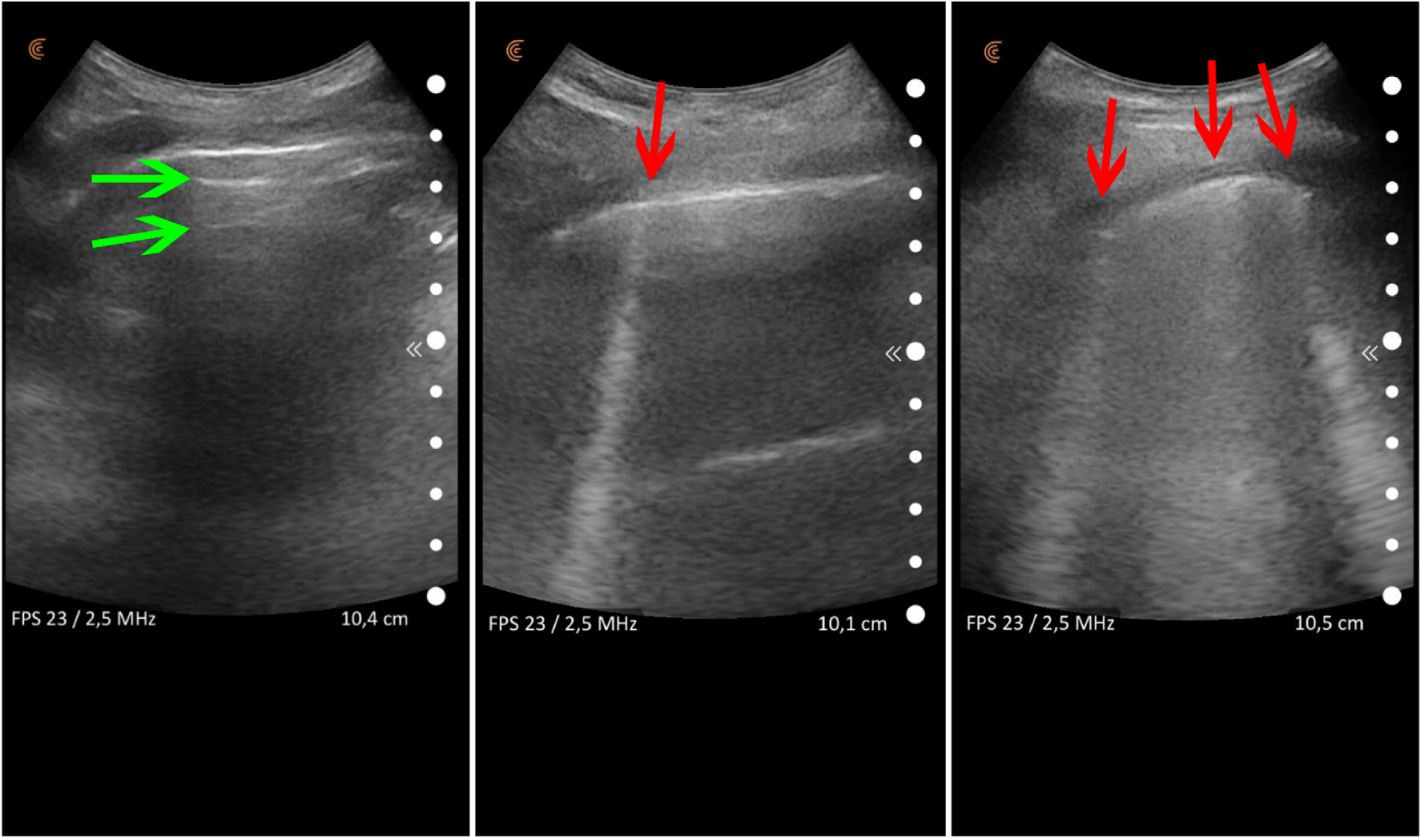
Acoustic artifacts in lung ultrasound: A‐lines (horizontal artifacts indicated by the green arrows) are the reverberation of the pleural line and suggest that the lung is aerated. B‐lines are vertical artifacts (indicated by the red arrows) that suggest a progressive decrease of lung aeration as the number of B‐lines increases.
